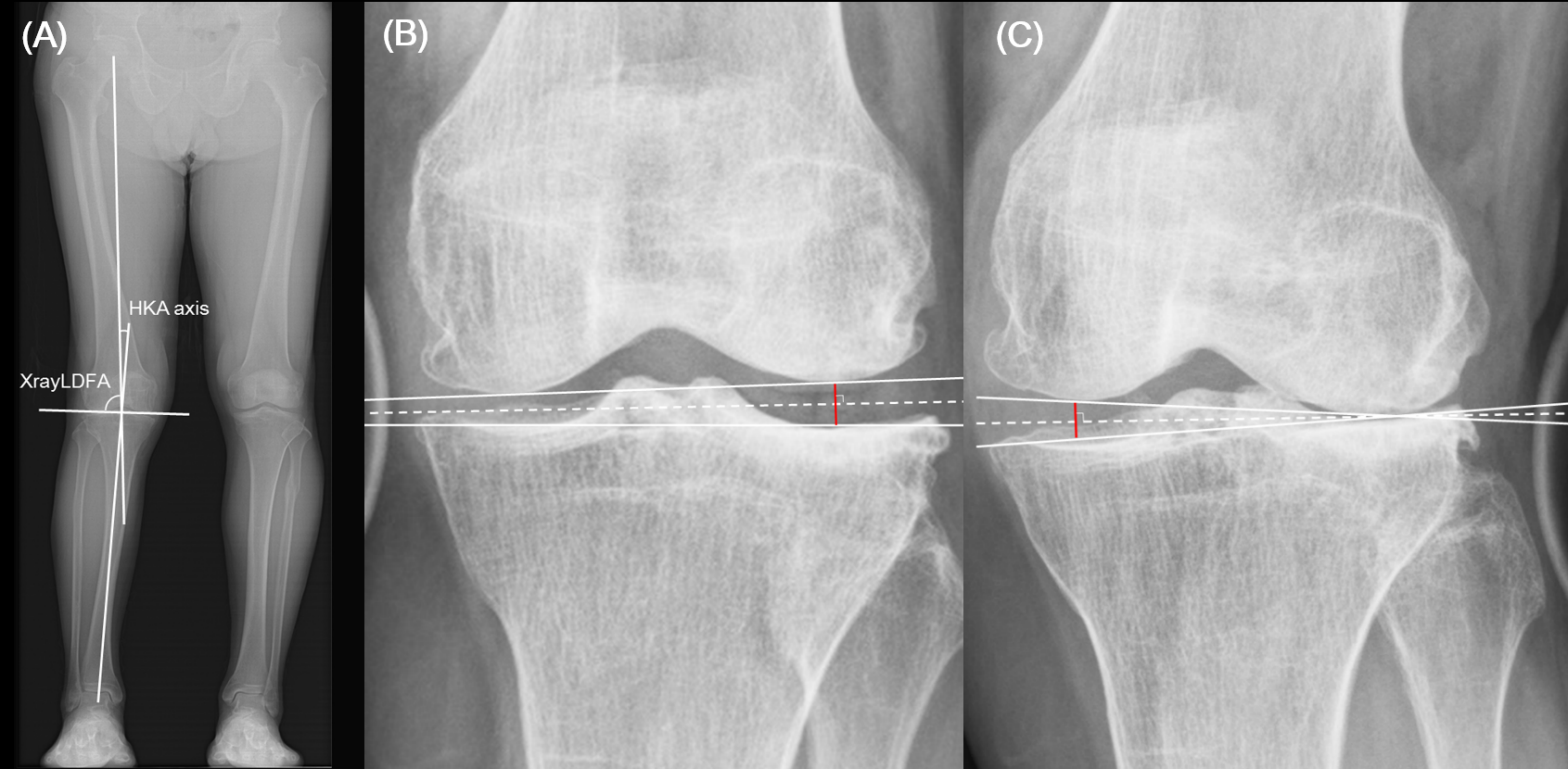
Measurement of HKA Axis, XrayLDFA, LGVS, and MGVS. The HKA axis and XrayLDFA were measured using a preoperative whole-leg standing radiograph (A). The HKA axis was measured using intersecting lines from the femoral and tibial mechanical axes. The XrayLDFA was a superolateral angle measured by intersecting the femoral mechanical axis line and distal femoral joint line. LGVS and MGVS were measured from varus (B) and valgus (C) stress radiographs, applying 130 N. The *dashed line* is the bisector of the angle between the distal femoral and proximal tibial joint lines (*black solid lines*). LGVS was measured from the lowest point of the lateral femoral condyle to its corresponding point on the tibial joint line (*red solid line*, drawn perpendicular to the *dashed line*). MGVS was measured using a similar method.
